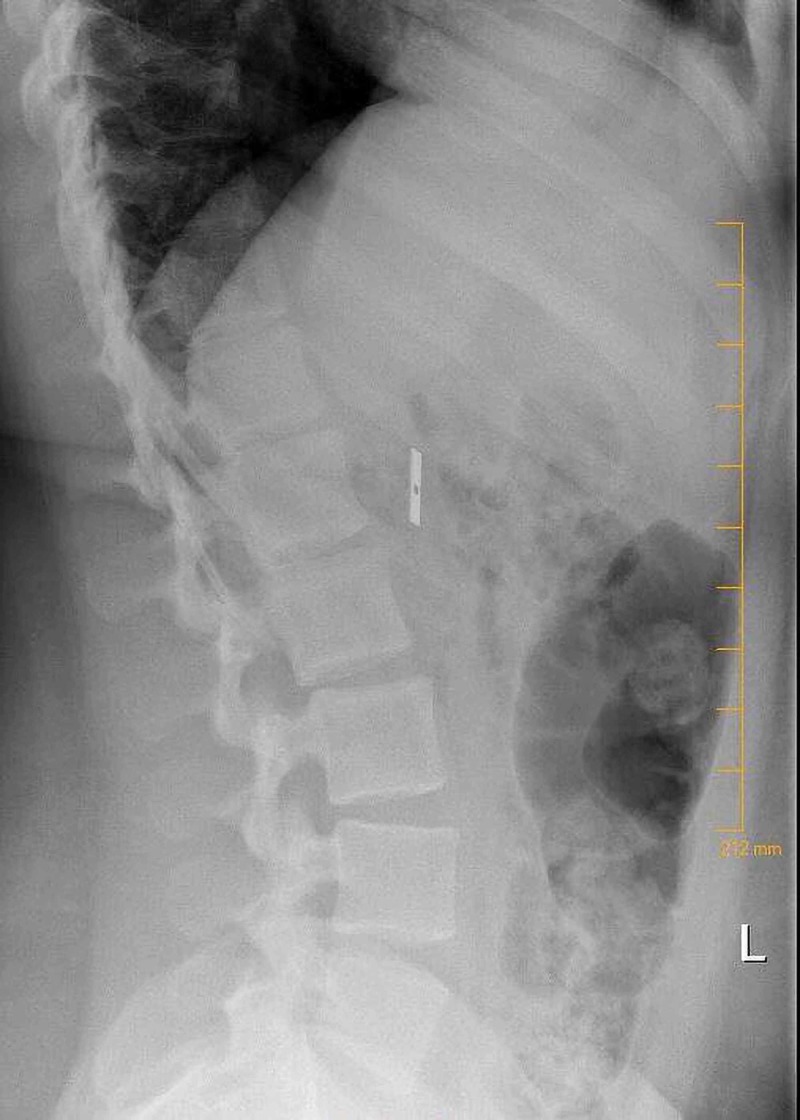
Lateral radiograph demonstrates an isolated metallic object in the abdomen consistent with suspected razor blade ingestion.
